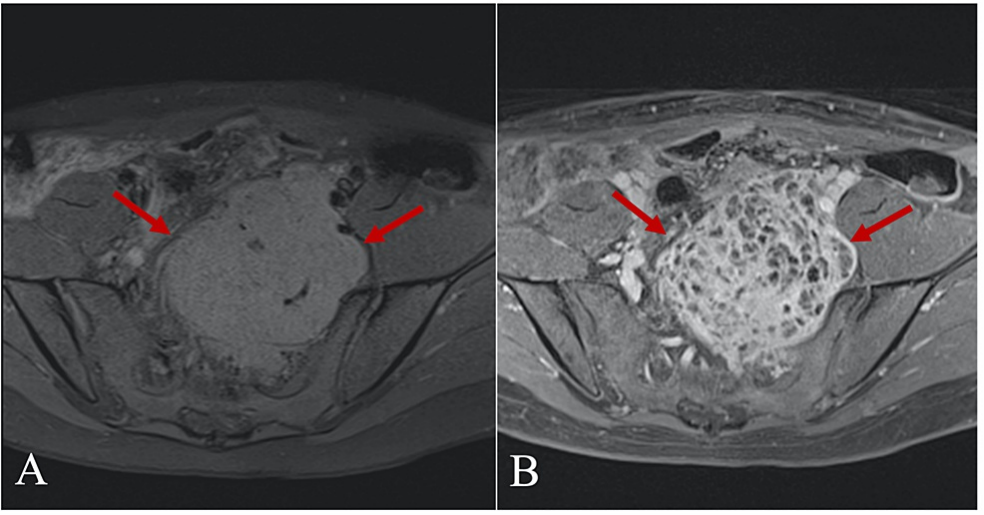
T1-weighted fat-saturated axial (A) and contrast-enhanced axial (B) MRI images. Huge sacral and presacral heterogeneous intermediate signal intensity tumor showing an avid post-contrast enhancement (arrows). No signs of infiltration to adjacent soft tissues can be seen. MRI, magnetic resonance imaging
